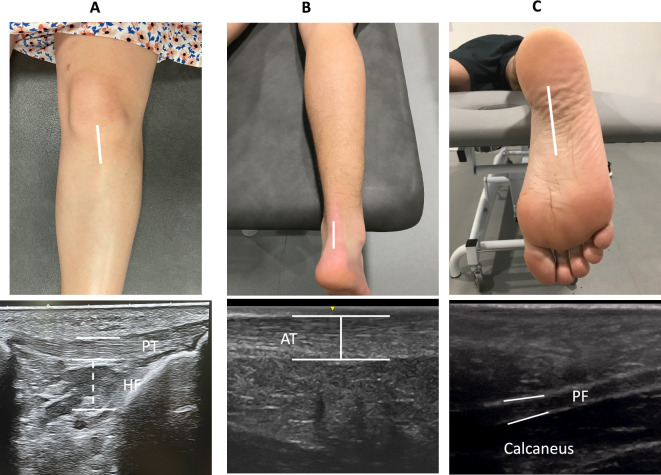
Ultrasound assessment of Hoffa’s pad, achilles tendon and plantar fascia thickness.
